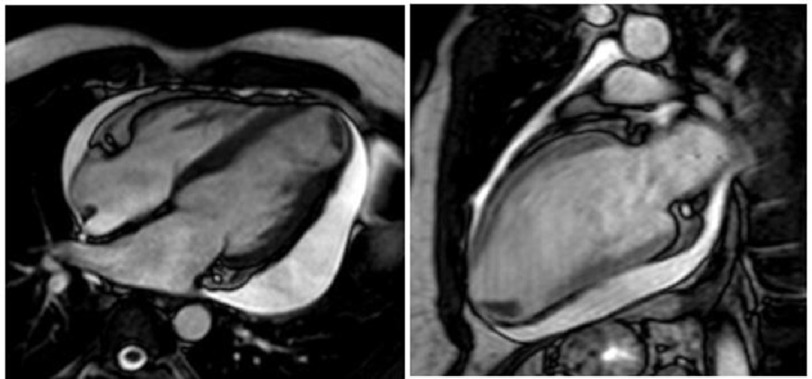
Cardiac MRI revealed a left apical aneurysm with transmural infarction and a left apical thrombus. Epicardial delayed myocardial enhancement in several regions was evident.
